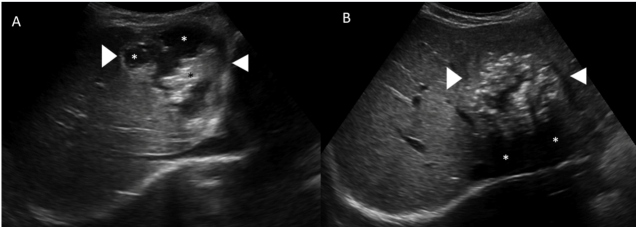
Transverse ultrasonography images (A, B) show a heterogeneous mass lesion (white arrowheads) that contained cystic components (A, white asterisks) and hyperechoic foci, representing calcifications (A, black asterisk; B, white asterisk: shadows of the calcifications).
